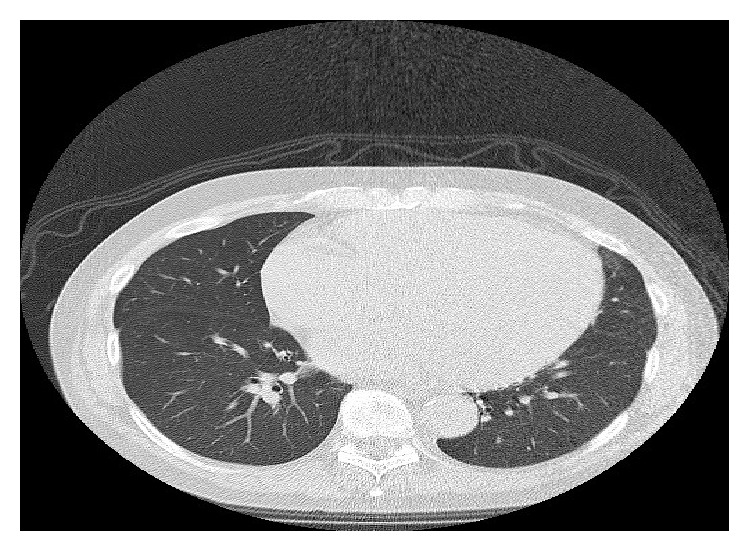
CT scan showing complete remission.
